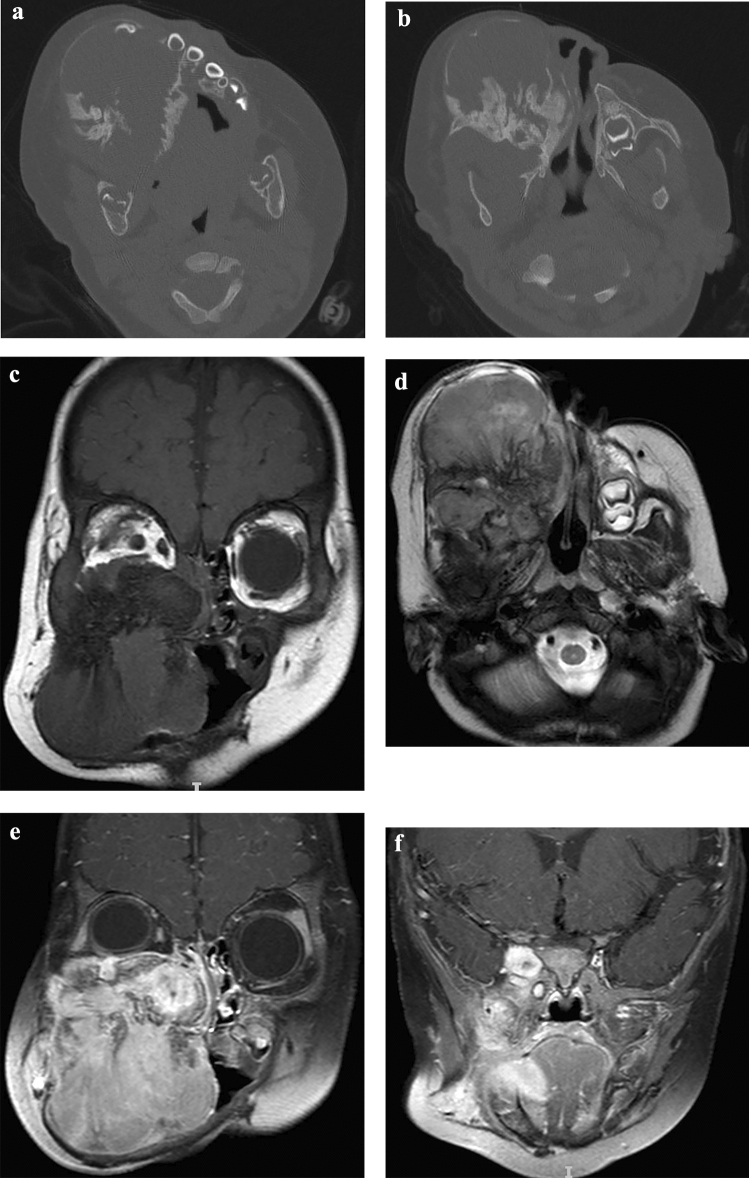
Case 3. a/b axial CT showed expansive tumor with obscure boundary, leading to extensive bone destruction, local spiculated periosteal reaction and fragmentary cortical bone. Several displaced/ “free-floating” teeth were distinct. c coronal T1 weighted image showed isointense or lightly hypo-intense signal. d axial T2 weighted image showed lightly hyper-intense signal. e/f coronal enhanced T1-weighted images showed intense but inhomogeneous enhancement following gadolinium injection. c/d/e/f Right maxilla, infratemporal fossa, pterygopalatine fossa, orbital apex and cavernous sinus were involved. Right orbit was smaller than the contralateral side causing by the compression of neoplasm. Nasal septum was also compressed tipping to left side
